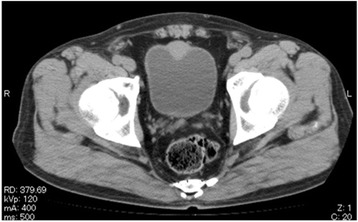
Computed tomography imaging findings of the bladder
